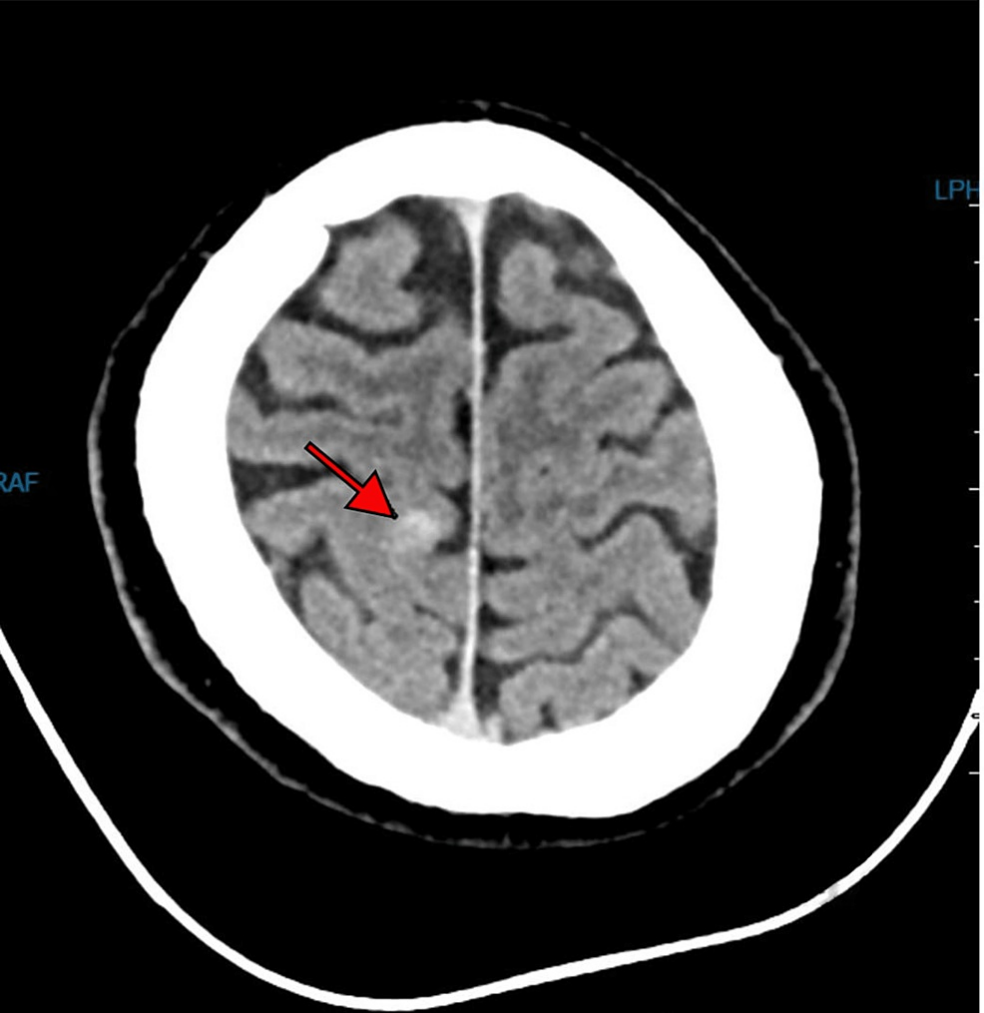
CT axial view: right parietal hemorrhagic lesion
